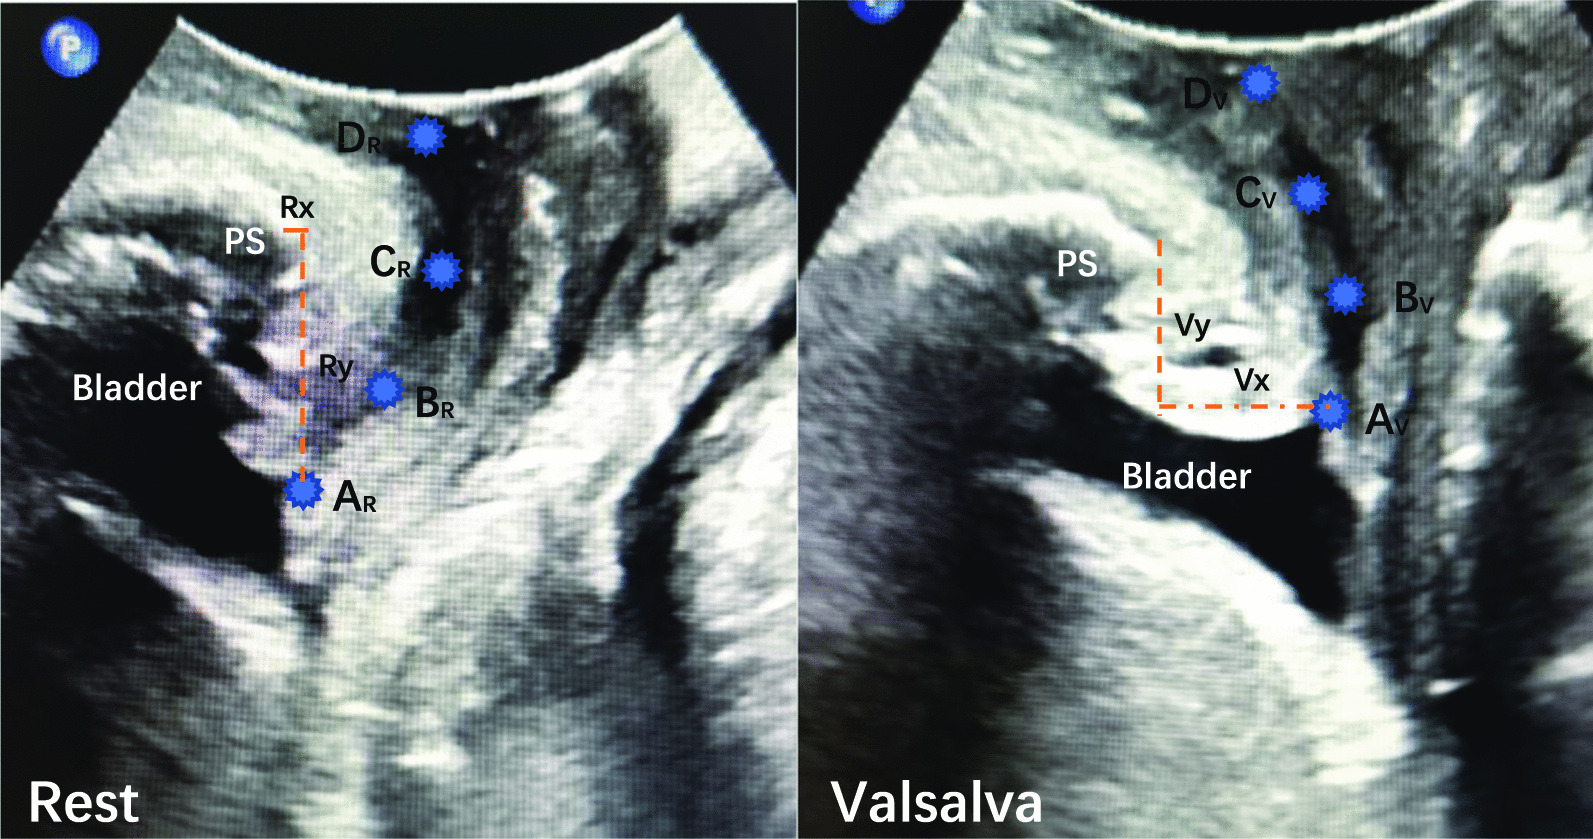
The urethra image was measured by perineal ultrasound image in the mid-sagittal plane at rest (left) and on Valsalva (right). The urethra is divided into three segments, defined by four points from the bladder neck (point A) to the external meatus (point D). The inferoposterior margin of the symphysis pubis serves as reference. The motion of Point A is shown as an example. A_R_ was the position of point A at rest, and A_V_ was the position of point A on maximal Valsalva manoeuver
